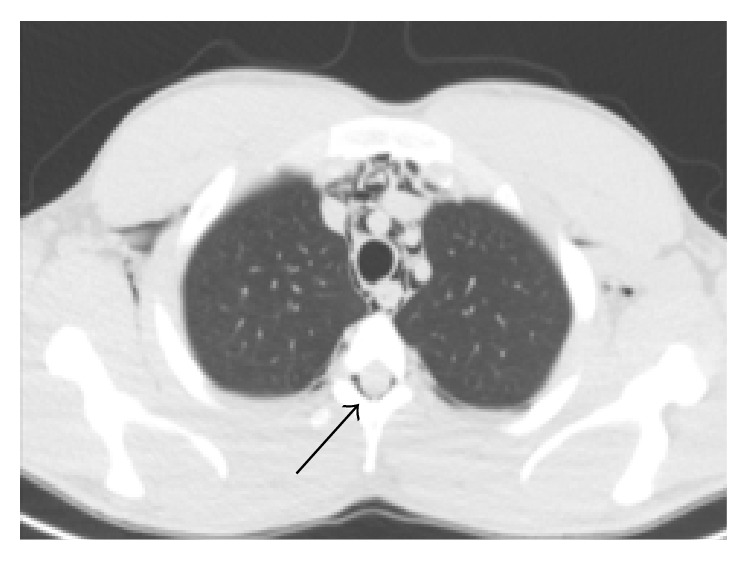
CT lung windows showing pneumomediastinum and pneumorrhachis (black arrow).
